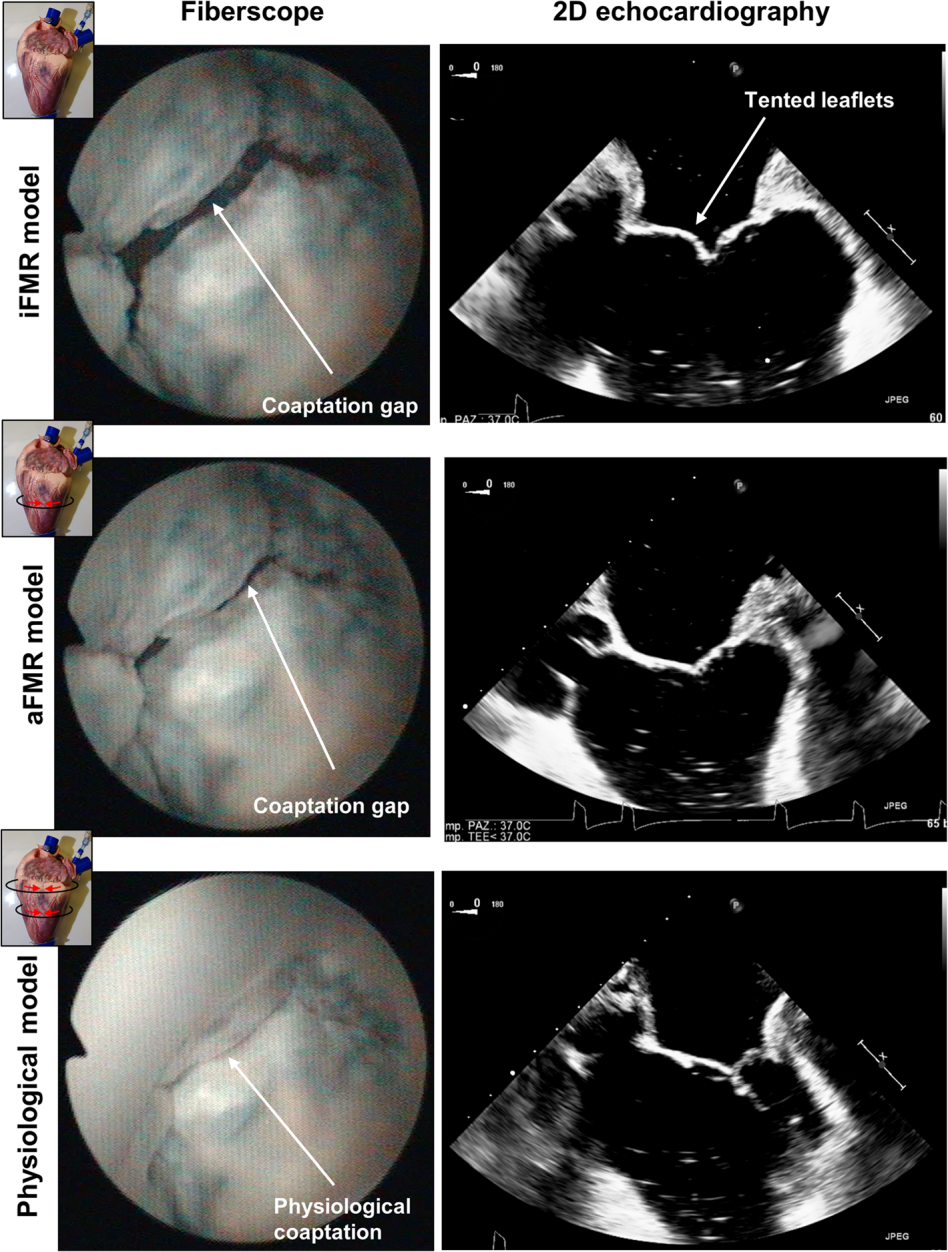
Characterization of the model. Exemplary mitral valve views from fiberscope (atrial view, left) and 2D echocardiography (3 chambers like view, right) in ischemic functional mitral regurgitation (iFMR), atrial functional mitral regurgitation (aFMR), and physiological model
